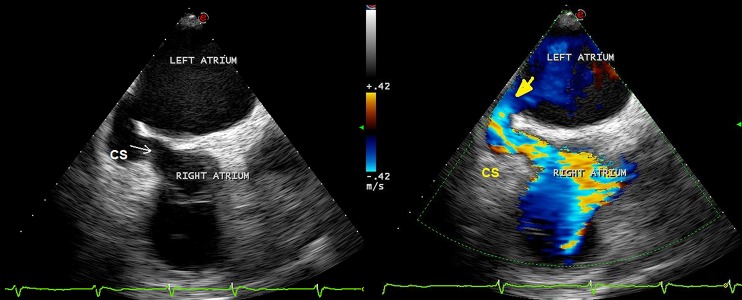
Transesophageal echocardiogram at 120 degree demonstrating the connection (yellow arrow) between the mid portion of coronary sinus and left atrium (left). Color flow Doppler revealed left to right shunt (right) Abbreviation: CS: Coronary Sinus; LA: Left Atrium
